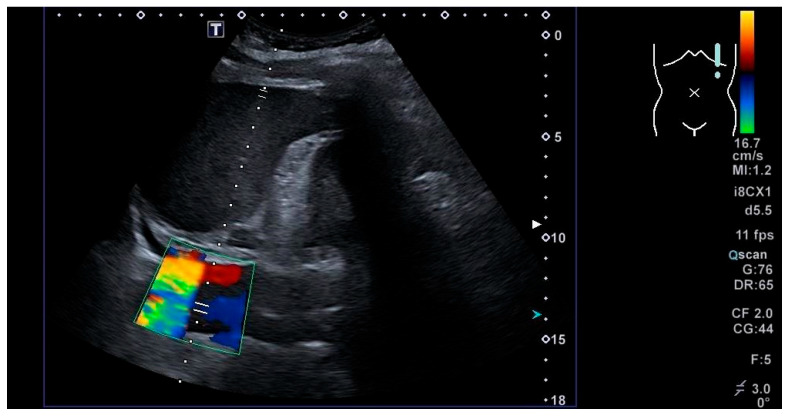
Patient’s echocardiogram.
